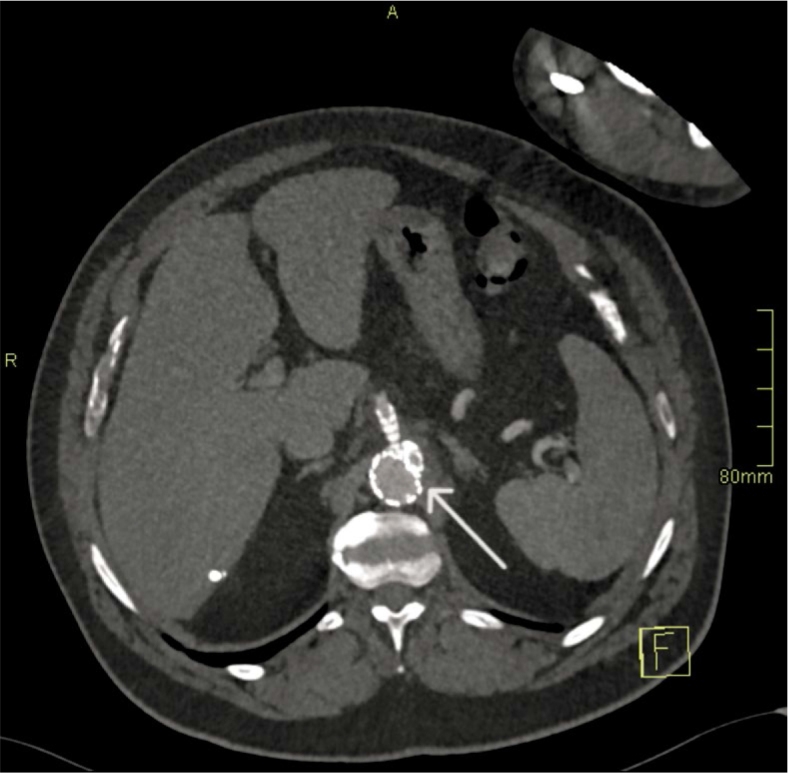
Follow-up after complex endovascular aortic repair (EVAR). Axial CT angiography (CTA) demonstrates a stable excluded sac without endoleak.
